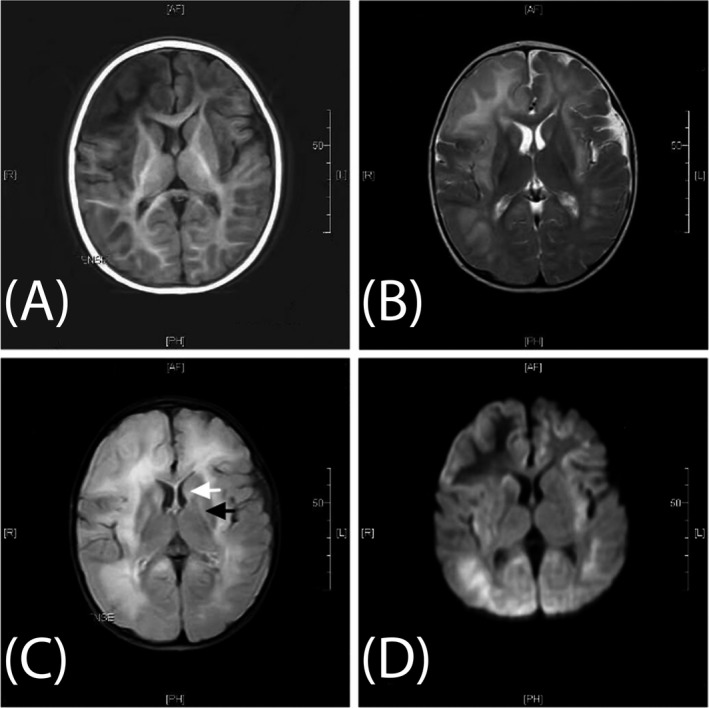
Brain magnetic resonance imaging of Patient 4 upon initial acceptance into the study. Brain MRI examinations were all performed at 1.5 T at the Children's Hospital of Chongqing Medical University. Axial T1 image (A), T2 image (B), fluid‐attenuated inversion recovery image (C), and diffusion‐weighted image (D) show diffused parenchymal edema. (A) Low signal intensity on T1‐weighted image (T1WI). (B) High signal intensity on T2‐weighted image (T2WI). (C) High signal intensity on FLAIR image involving the white matter, caudate head (white arrow), and bilateral putamina (black arrow). (D) High signal intensity on diffusion‐weighted image (DWI)
